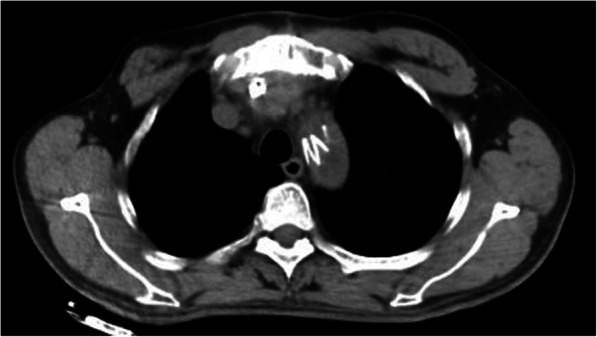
CT scan showing a little contrast agent surrounding the displaced aortal arch with a bit of gas
